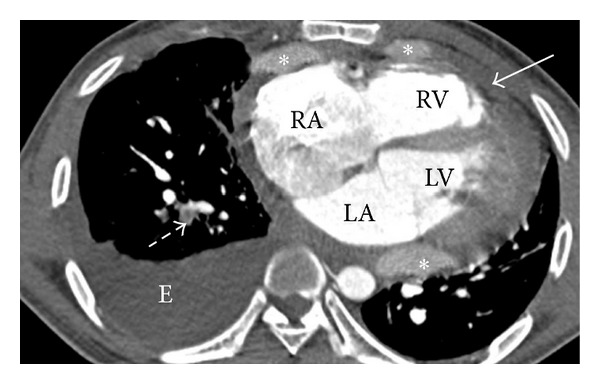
Axial contrast-enhanced CT scan showed enhanced soft tissue nodules (asterisk) that have infiltrated the pericardium, moderate amount of pericardial effusion (arrow), right pleural effusion (E), and partially occlusive clot in posterobasal segmental branch of right lower lobe pulmonary artery (dashed arrow) (RA: right atrium, LA: left atrium, RV: right ventricle, LV: left ventricle).
